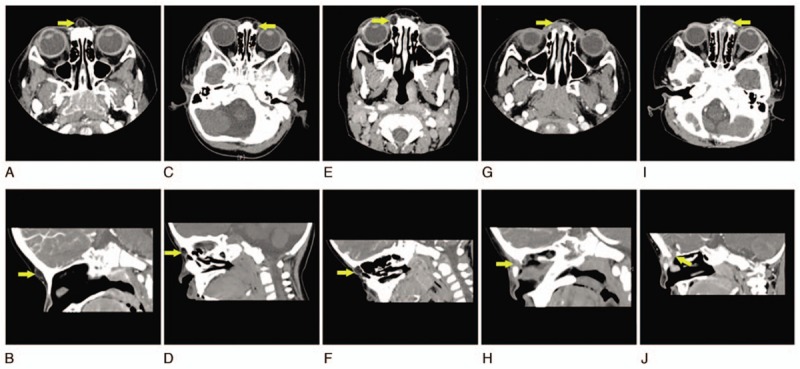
Computed tomography (CT) findings of each type among patients in this study. A and B, A type I lesion on horizontal and sagittal CT in the same patient. C and D, A type II lesion in 1 patient. E and F, A type II lesion in another patient. G and H, A type III lesion. I and J, A type IV lesion. The relationships of the lesion, nasal bone, and anterior skull base can be clearly observed in the images.
